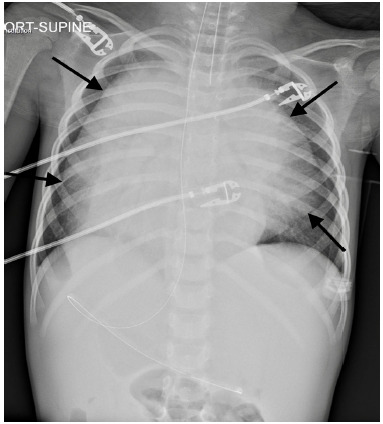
Supine portable frontal chest radiograph with arrows pointing to what resembles bilateral pneumothoraces.
